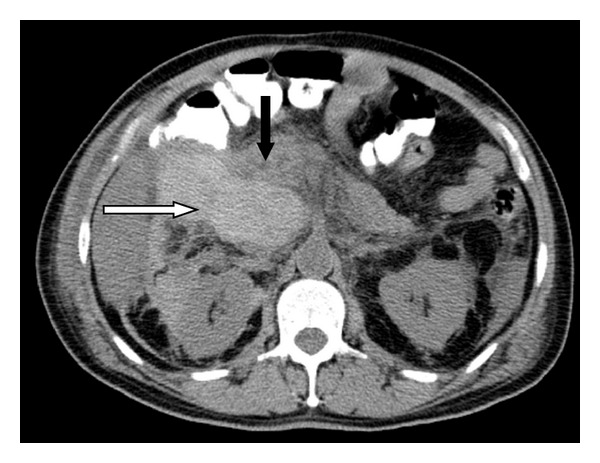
CT of abdomen revealed a large hematoma inferior and medial to the liver (white arrow) and abutting the pancreatic head (black arrow).
